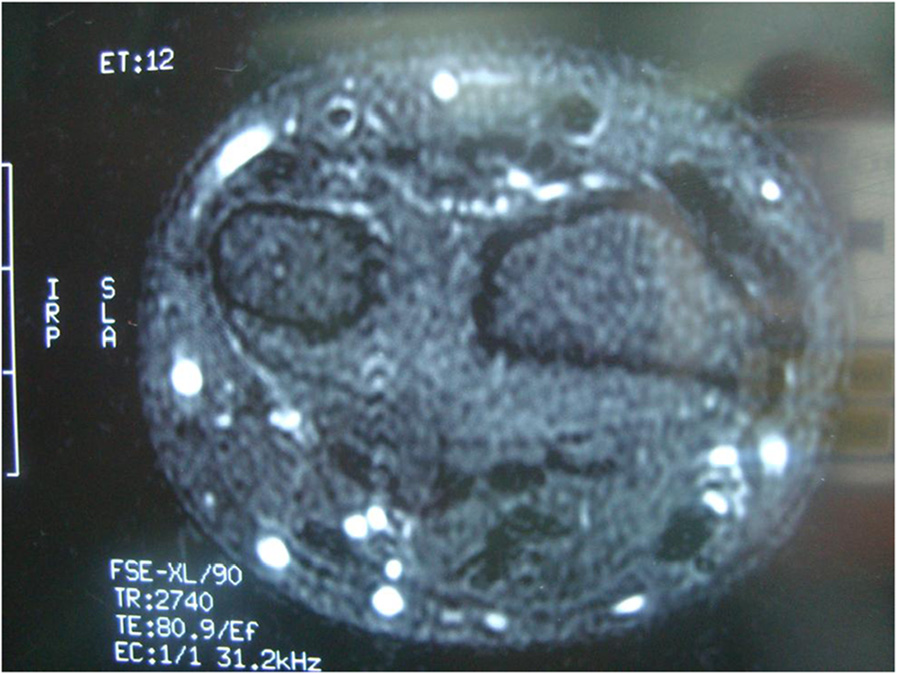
T2 magnetic resonance images; extensor carpi ulnaris tendon with inflammation around sheath.
